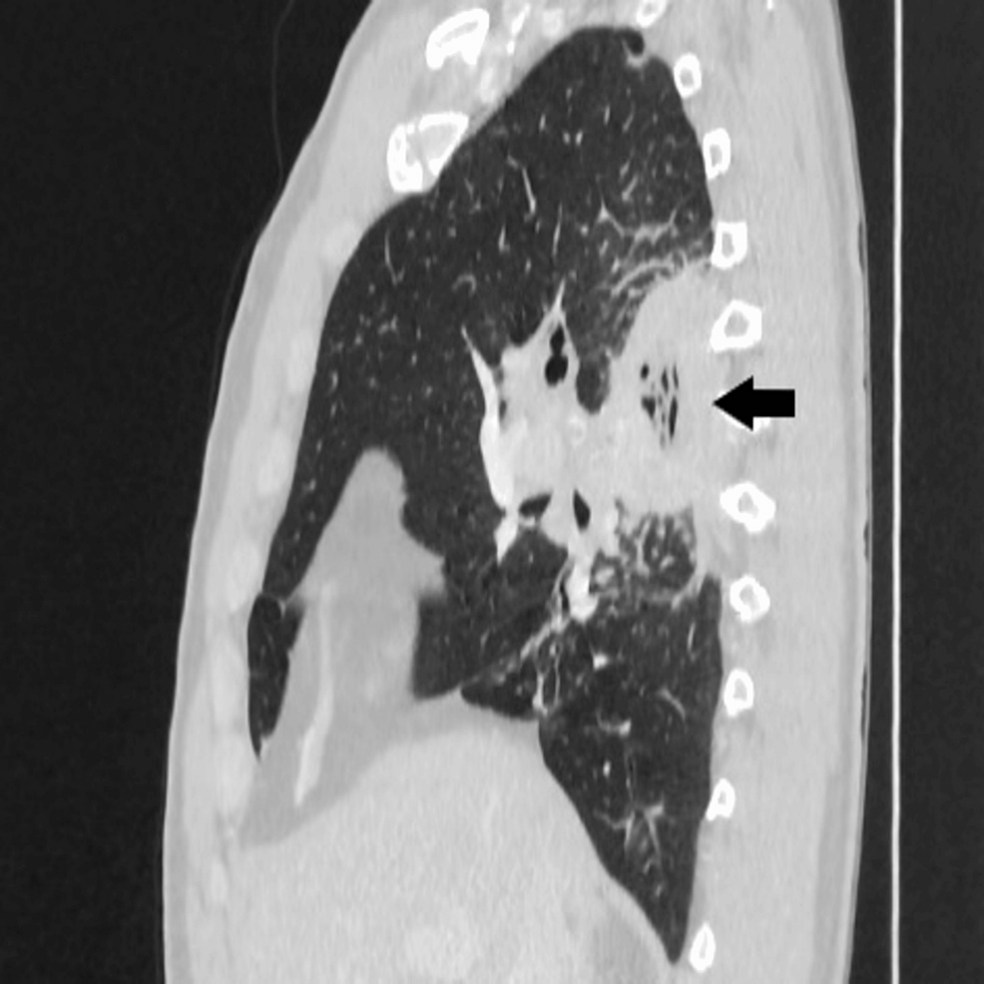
Computed tomography. Sagittal images of lung abscess (black arrow).
